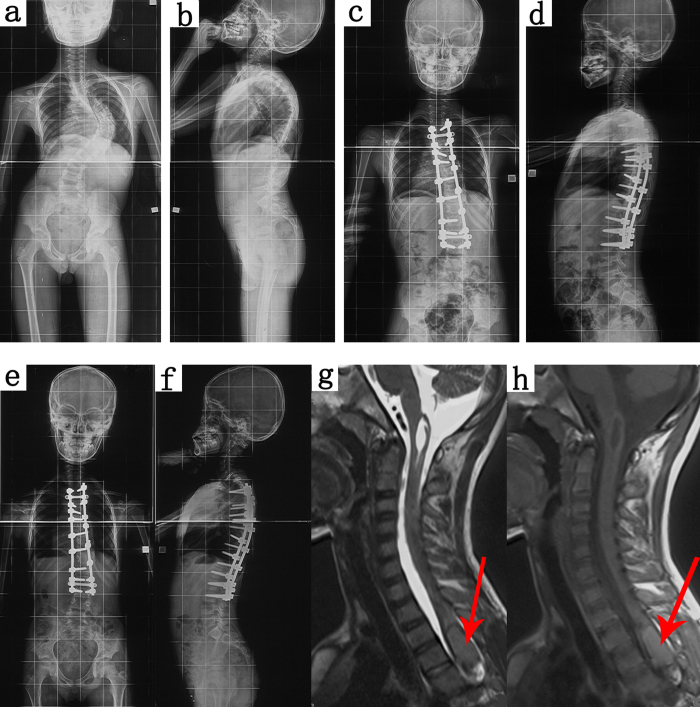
Images of an 11-year-old boy with congenital scoliosis associated with intraspinal mass. The patient underwent single-stage intraspinal mass resection and scoliosis correction. He experienced transient neurologic deficit postoperatively, but recovered to normal 6 months later. (a,b) Preoperative radiographs, demonstrating a left curve of 85° and kyphosis of 66°. (c,d) Postoperative radiographs, demonstrating a left curve of 18° and kyphosis of 36°. (e,f) Final follow-up radiographs, demonstrating a left curve of 26° and kyphosis of 42°. (g,h) Magnetic resonance images, demonstrating an intraspinal mass at T2–7.
